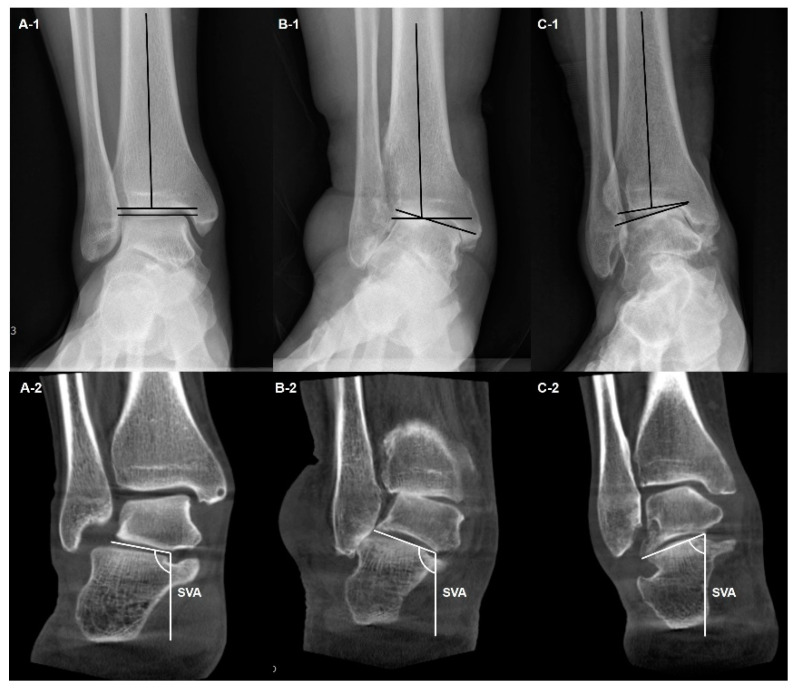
Weight-bearing plain radiographs of patients with various types of tibiotalar alignment (A-1,B-1,C-1) and corresponding subtalar joint orientation in WBCT (A-2,B-2,C-2). The subtalar vertical angle (SVA) was defined as the inclination of the line connecting the medial and lateral aspects of the talus with a vertical line, which is perpendicular to the ground.
